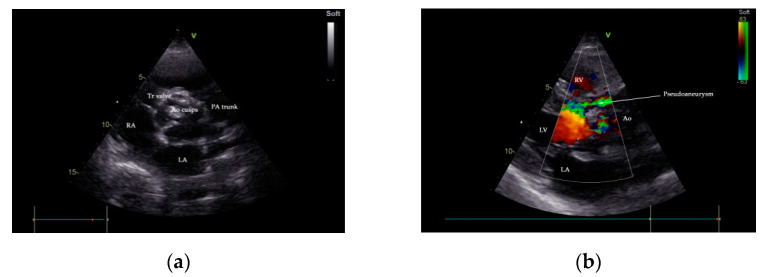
Transthoracic echocardiography (TTE), parasternal short-axis-base view, showing a heavily calcified aortic valve with possible vegetation (a); communication between LVOT and P-MAIVF is shown by color flow Doppler, parasternal-long axis view (b). Ao—aorta, LA—left atrium, LVOT—left ventricular outflow tract, PA—pulmonary artery, P-MAIVF—pseudoaneurysm of the mitral-aortic intervalvular fibrosa, RA—right atrium, RV—right ventricle, Tr—tricuspid valve.
